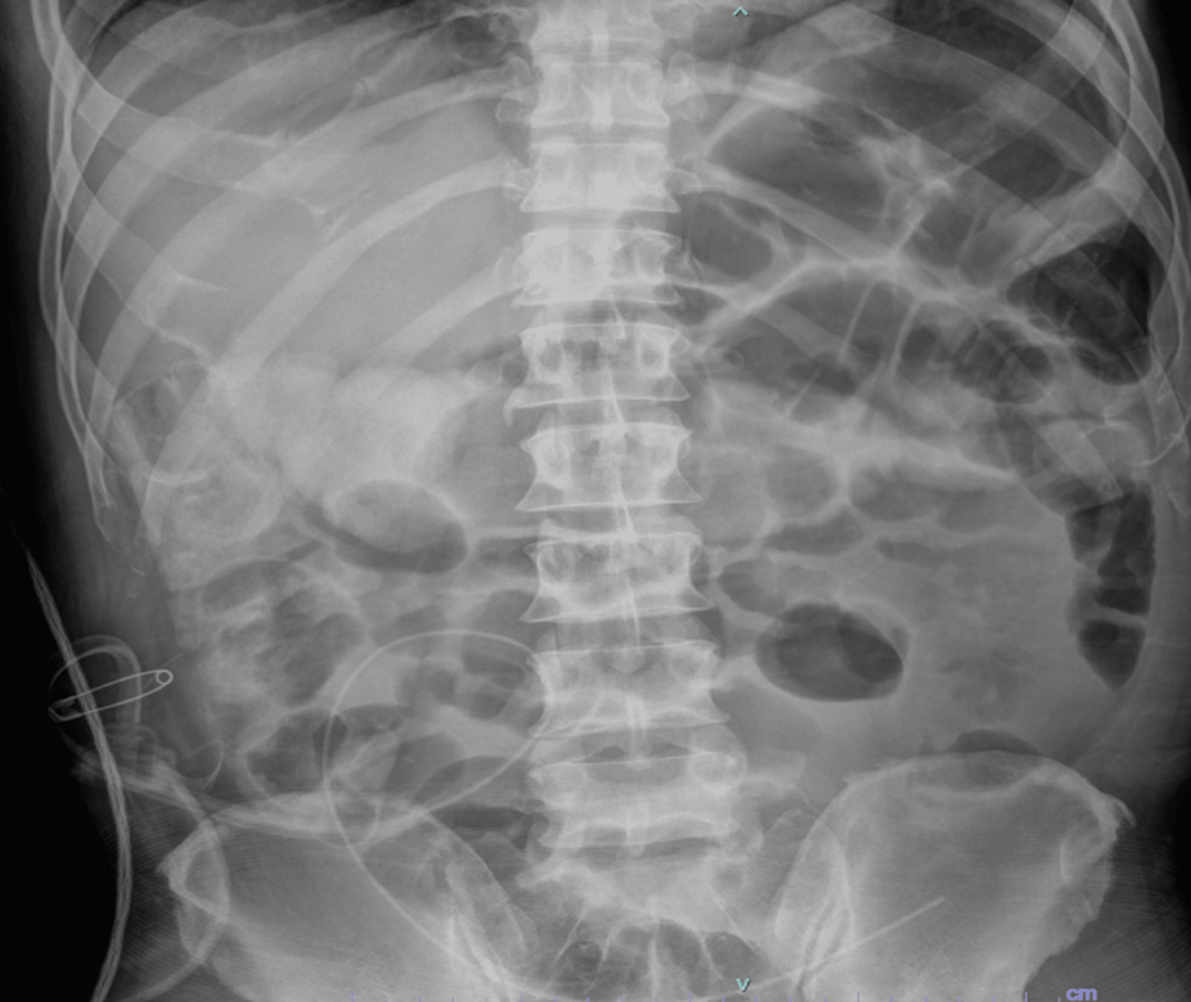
Abdominal radiograph showing persistently dilated small-bowel loops with residual contrast in the colon, suggestive of incomplete transit and ongoing small bowel obstruction.
